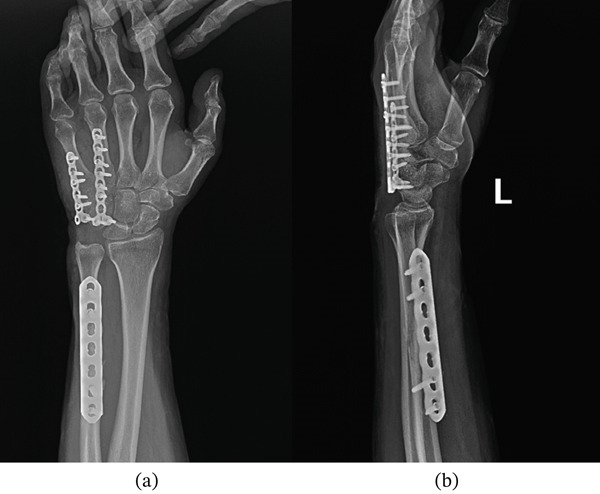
(a) Postoperative anteroposterior radiograph. (b) Postoperative lateral radiograph.
